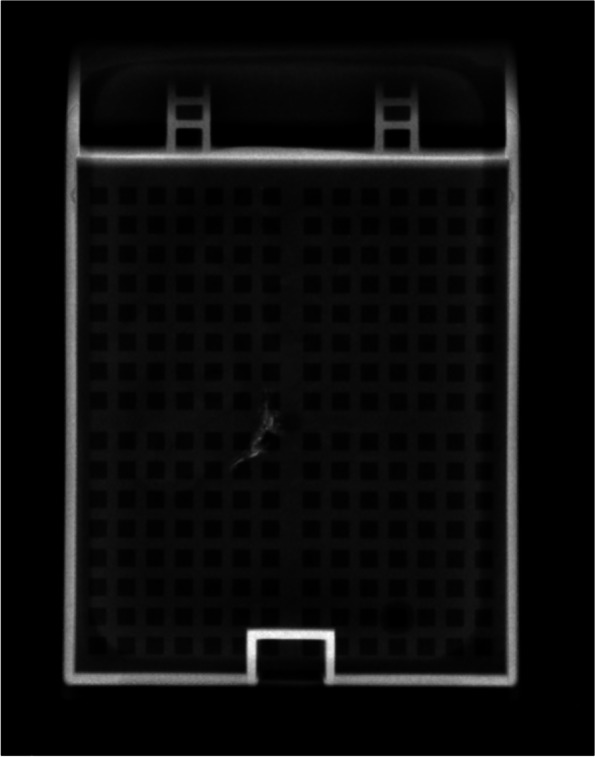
Radiograph of paraffin block showing opaque material in a case of barium sulfate deposition in the colon
